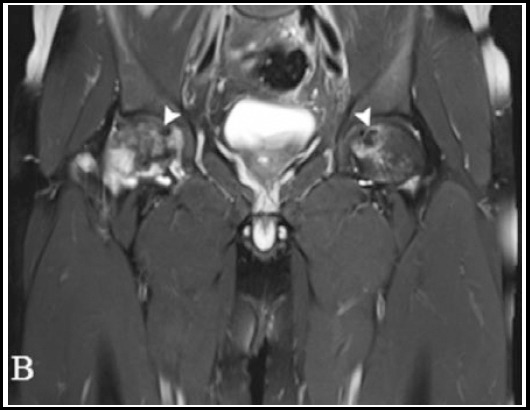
Coronal T2-weighted MR image of both hips shows well-demarcated areas of osteonecrosis (arrowheads) in the both femoral heads and bone marrow oedema in the right femoral heads.
